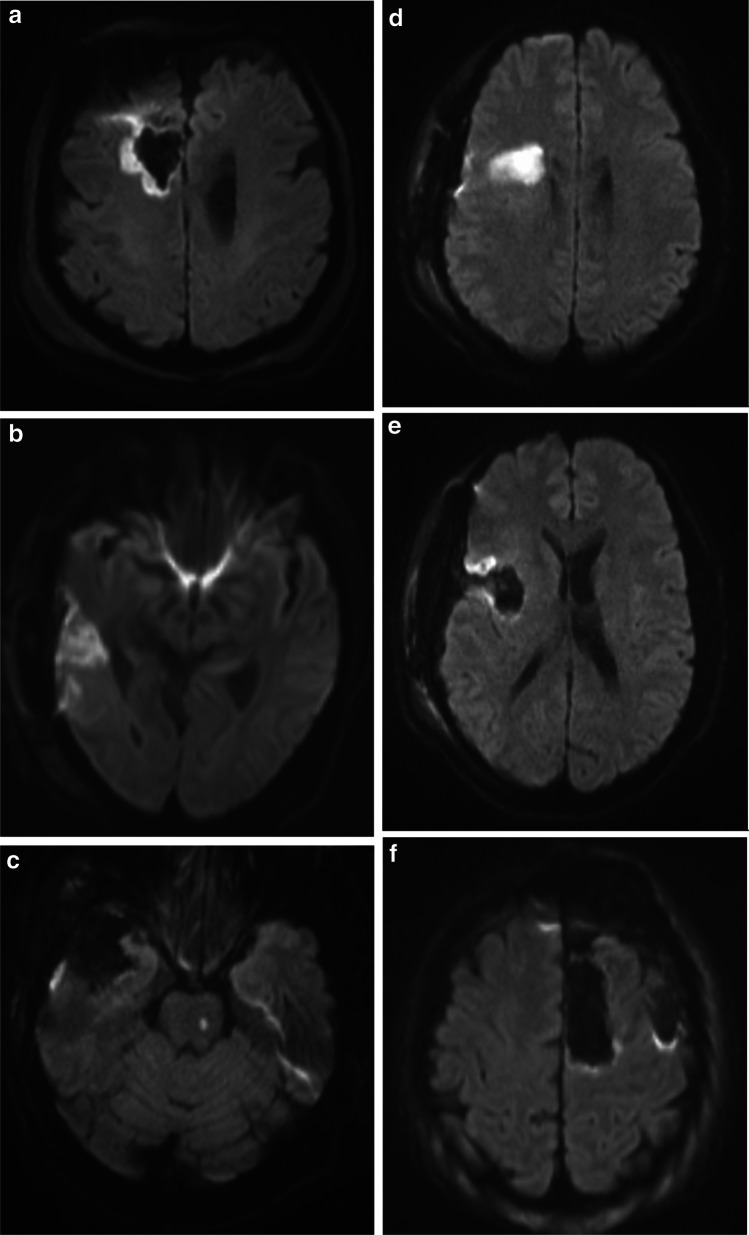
A Rim-shaped DWI change (volume = 2.7 cm^3^). B Sector-shaped DWI change (volume = 2.5 cm^3^). C A remote pontine DWI-change (volume = 0.2 cm^3^). D and E A sector-shaped and rim-shaped DWI change in the same brain (volume = 5.4 cm^3^). F A cavity without any significant DWI abnormality
